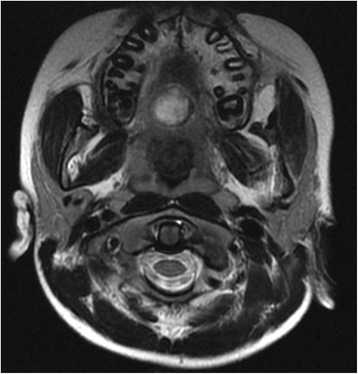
Contrast-enhanced magnetic resonance imaging showing the well-encapsulated lesion with thinning of the floor of the nasal cavity
